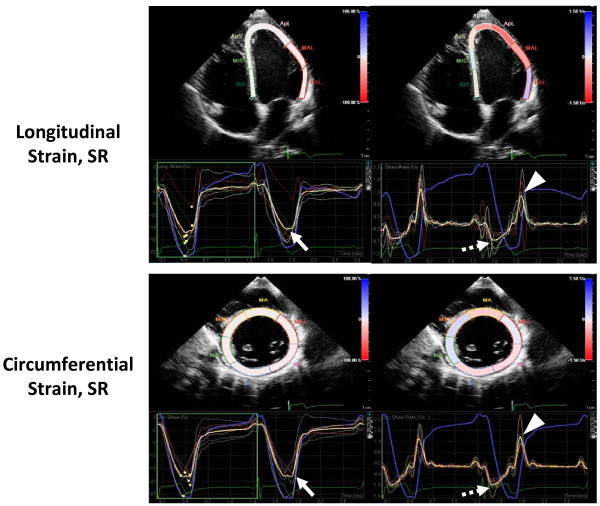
Example of an analysis using speckle-tracking echocardiography. Longitudinal and circumferential strain (*left panels*) and SR (*right panels*) curves are shown. Mean values of peak systolic strain (*solid arrow*), systolic SR (*broken arrow*), and early diastolic SR (*arrowhead*) are indicated.
